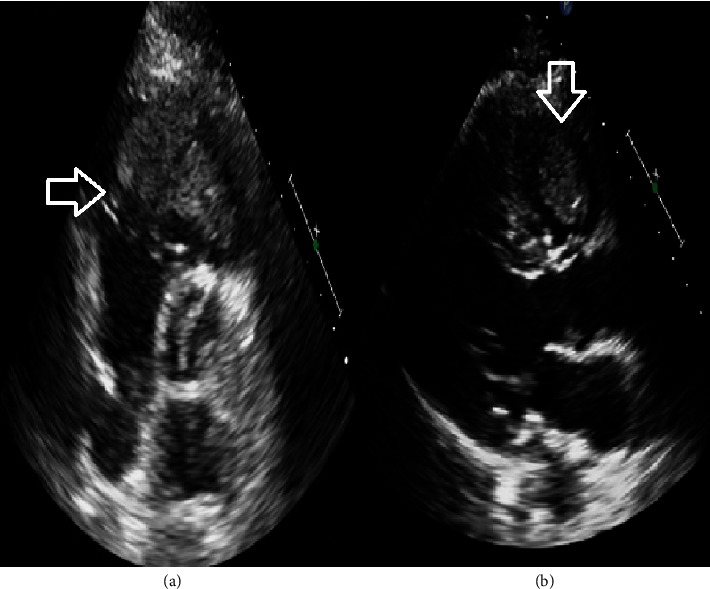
Transthoracic echocardiogram showing RV mass. (a) Parasternal short axis view demonstrates the mass (white arrow) extending from the undersurface of the tricuspid annulus and traversing the pulmonic valve. (b) Redemonstration of RV mass (white arrow) in the parasternal long axis view. Abbreviations: RV: right ventricle.
